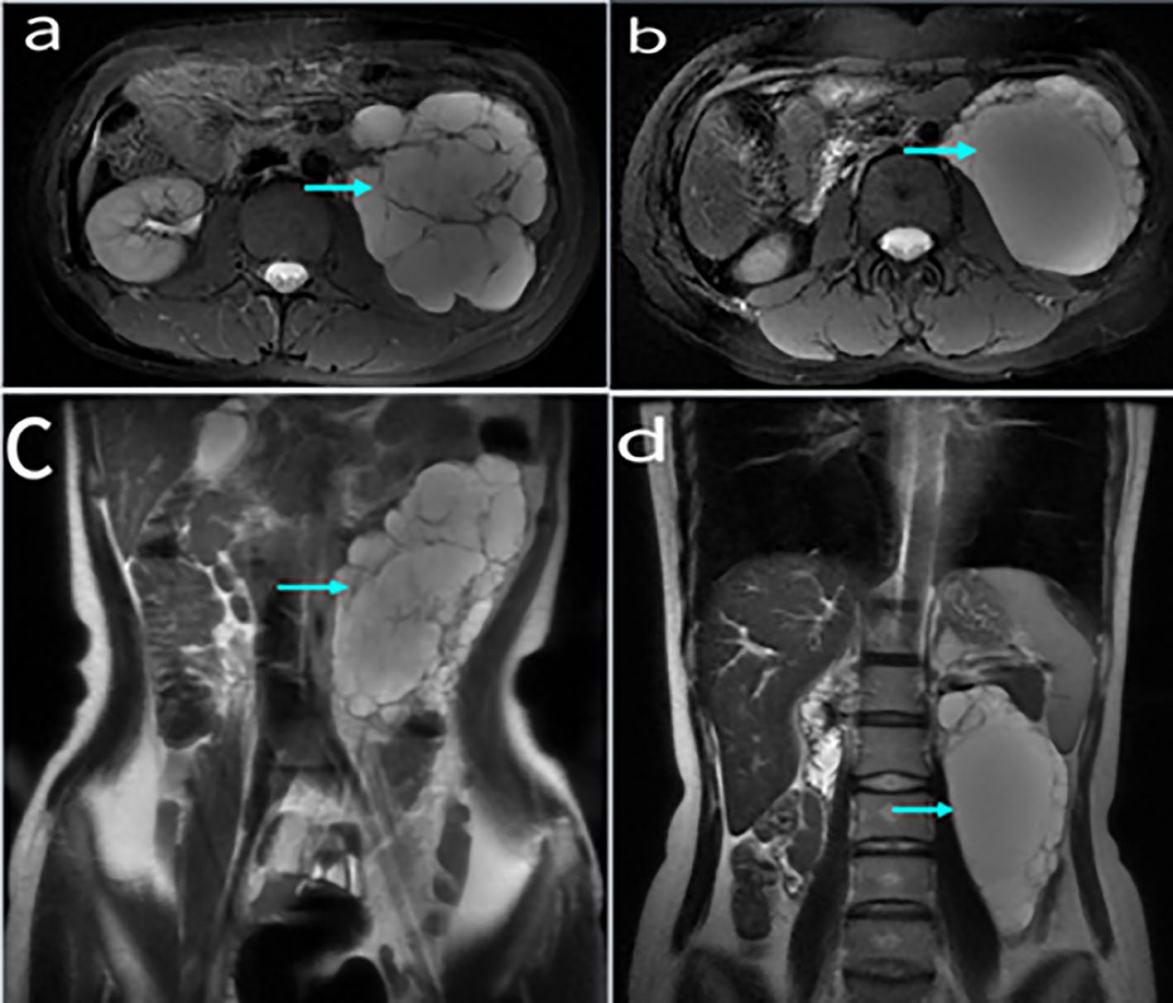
Enhanced MRI image of retroperitoneal lymphangioma
